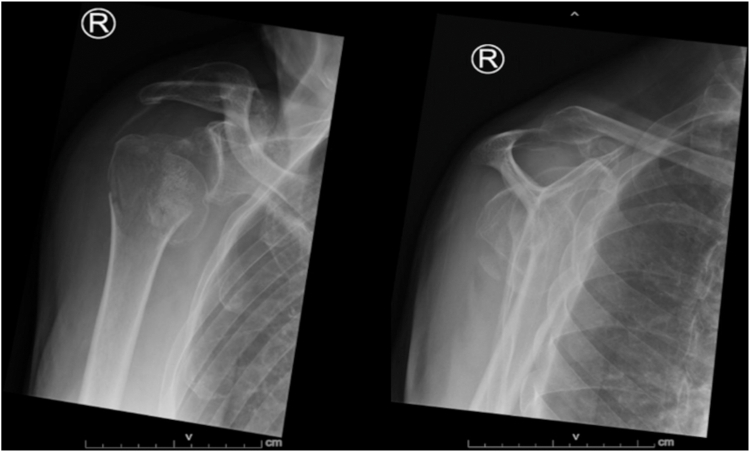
Anteroposterior (AP) (*left*) and lateral (*right*) preoperative plain radiographs of a complex four-part head split proximal humerus fracture. This case was used in the virtual surgical planning (VSP) for prospective use.
